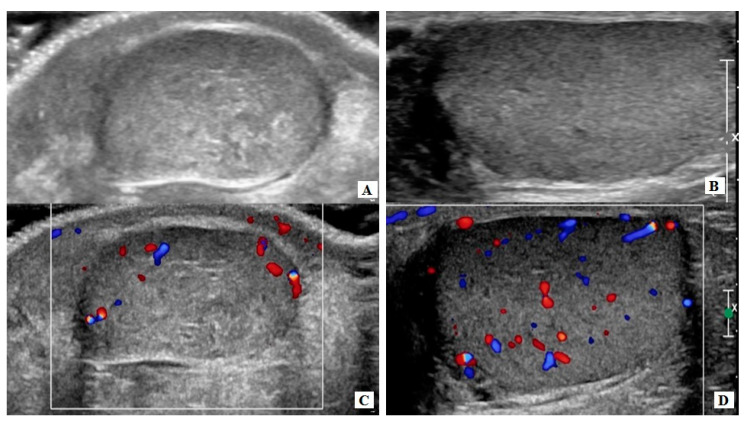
(A,B) No evidence of the lesion in B-mode and in color Doppler mode, immediately after surgery, (C,D) and in late follow up.
